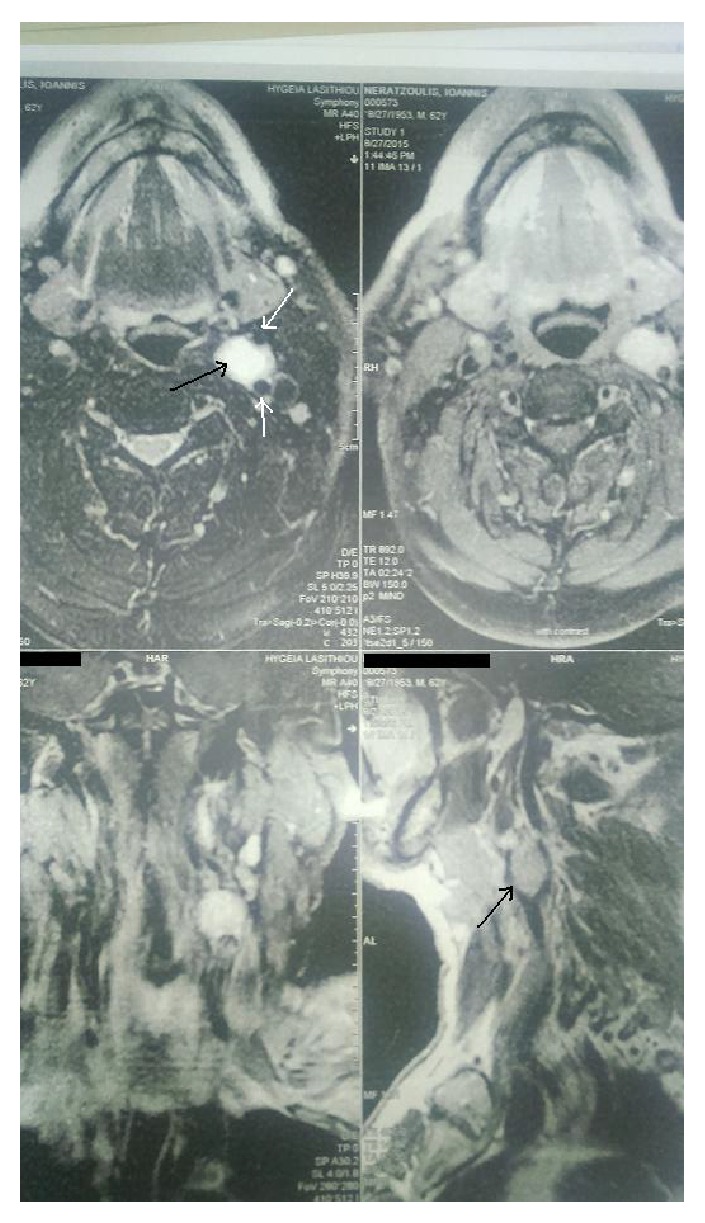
Magnetic resonance image showing a type II carotid body tumor (black arrows) that partially surrounds and compresses the carotid vessels (white arrows).
